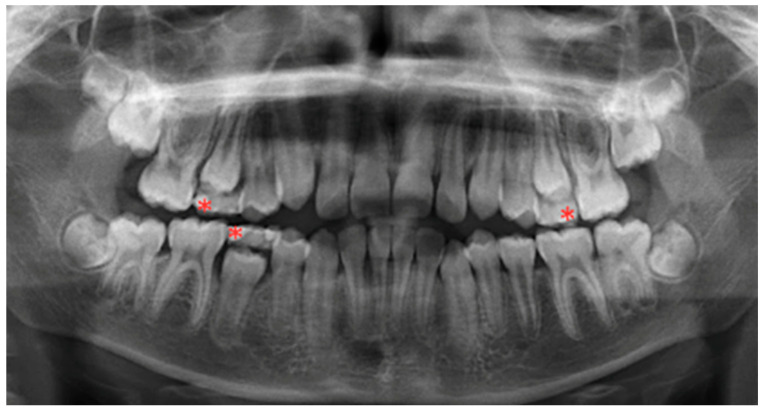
Orthopantomograph of a child in mixed dentition. * demonstrated primary teeth with the successor premolar in the Nolla’s stage 8; however, no alveolar crypt was observed, and teeth were not considered PPT.
